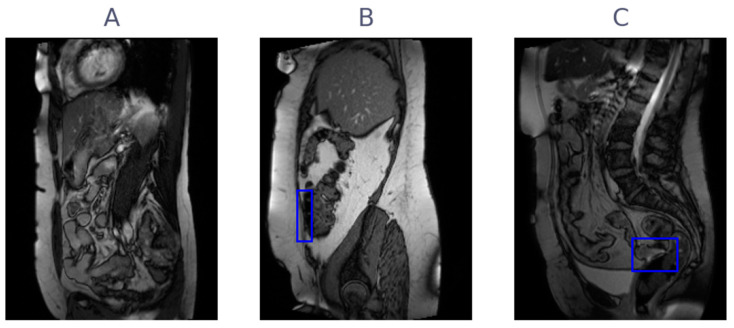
Three examples of cine-MRI slice with typical adhesions, with consensus annotations overlayed as blue boxes. (A) A slice without adhesions present, (B) a slice with an adhesion to the anterior abdominal wall, and (C) a slice with an adhesion in the pelvic area.
